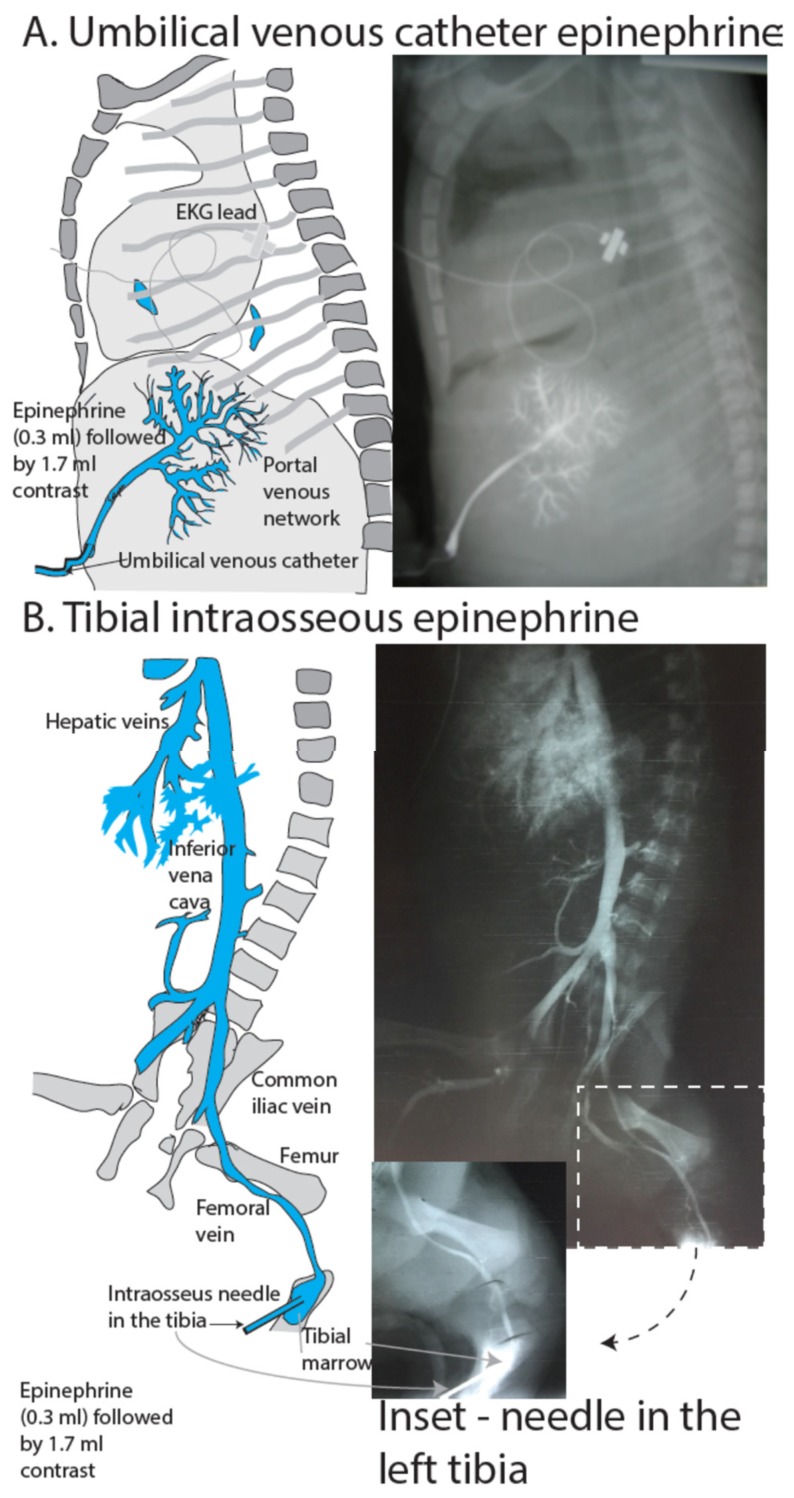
X-ray showing distribution following (A) low-lying umbilical venous catheter or (B) tibial IO administration of contrast in a perinatal cardiac arrested lamb model following 30 sec of chest compressions. Rapid distribution of contrast into the venous vasculature and heart can be appreciated following IO administration. IO: Intraossesus. Copyright Satyan Lakshminrusimha.
